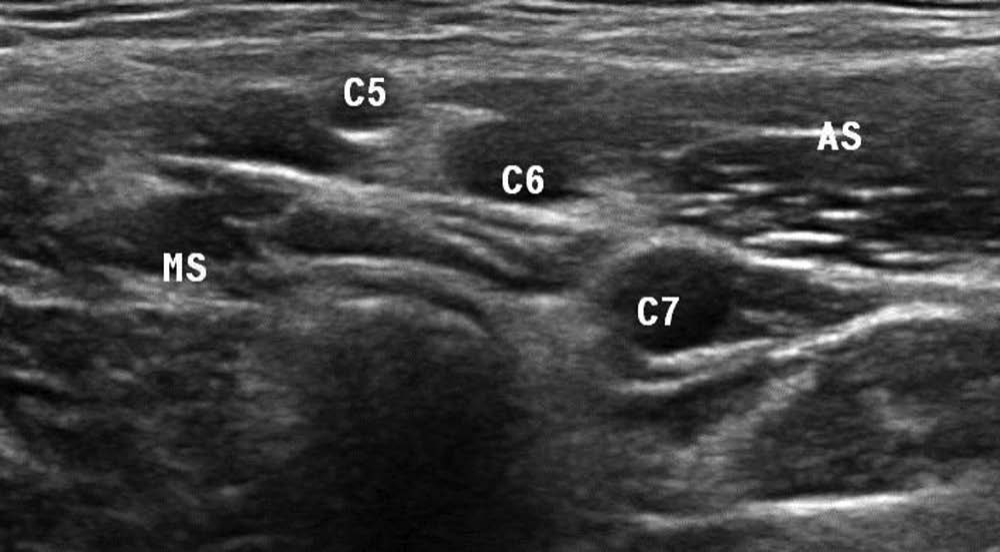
Short-axis sonographic image of the C5-C7 brachial plexus nerve roots, in the interscalene groove, in a patient with type 2 diabetes AS = Anterior scalene muscle, MS = middle scalene muscle.
